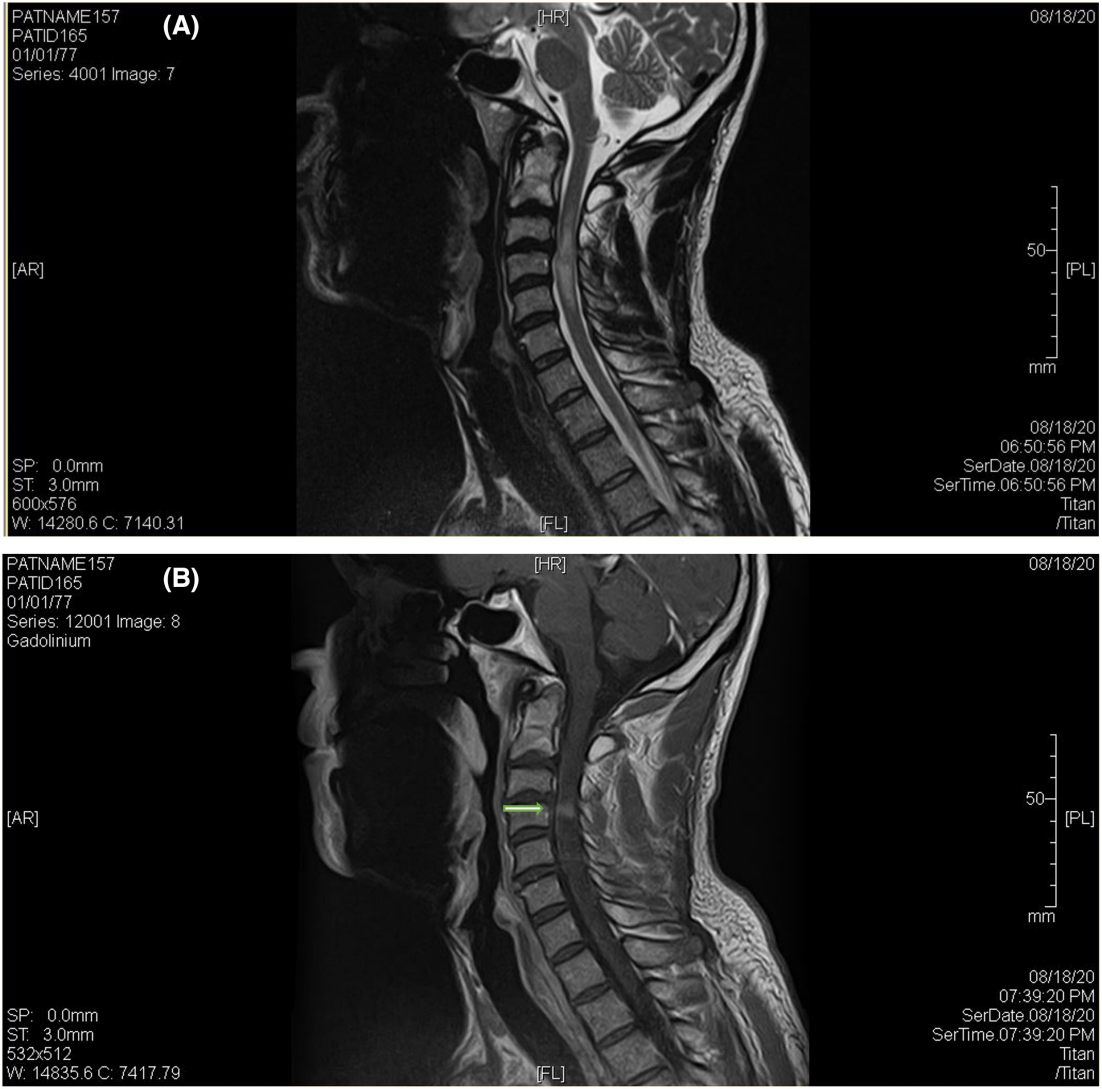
Neuroimaging of spondylosis with gadolinium enhancement. (A) Sagittal magnetic resonance imaging (MRI) reveals T2‐signal hyperintensity in the spinal cord. A long segment of cord edema is seen within the upper spinal cord from the C2–C3 disc space extending to the inferior endplate of C5. A disc bulge at C3–C4 mildly flattens the anterior aspect of the spinal cord. (B) A linear area of contrast with the “pancake enhancement pattern” is seen slightly below the disc level indicated by the arrows.
